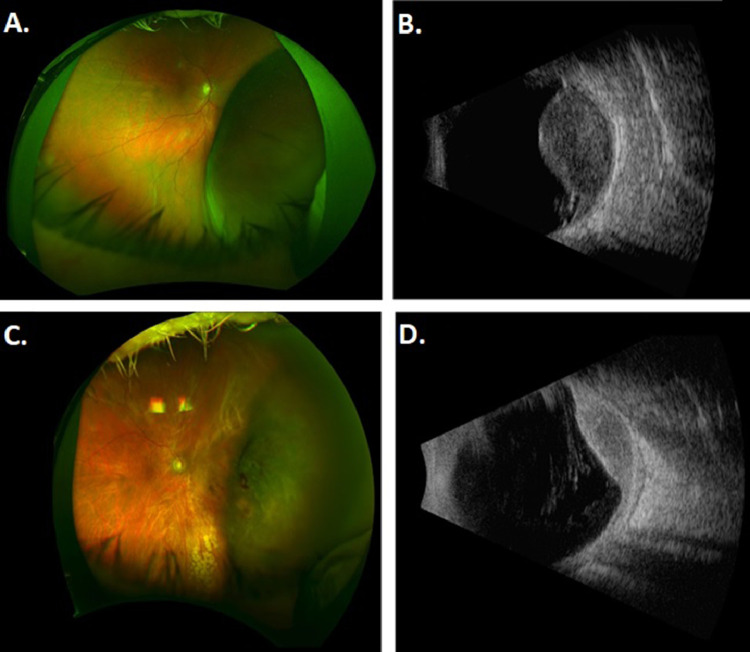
Tumor of patient A. (A) Fundoscopy at presentation. (B) Ultrasonography at presentation. (C) Fundoscopy at last follow-up. (D) Ultrasonography at last follow-up.
